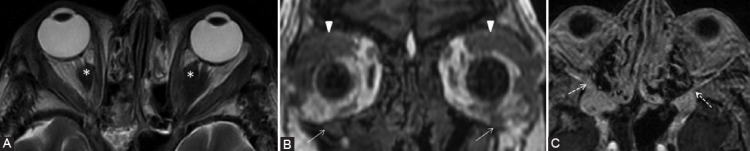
Same patient as in Figure 19. Axial T2-weighted (A), coronal T1-weighted (B), and axial contrast-enhanced 3D T1 (C) images. There are T2 hypointense focal masses (*) encasing both optic nerves. The infraorbital nerves are thickened, expand the infraorbital canal bilaterally (arrows), and show pathologic CE that extends to the inferior orbital fissure (dashed arrows) bilaterally. Involvement of the frontal nerves (branch of V1) is suggested by the bilateral fat obliteration (arrowheads) above the superior orbital levator muscle.
